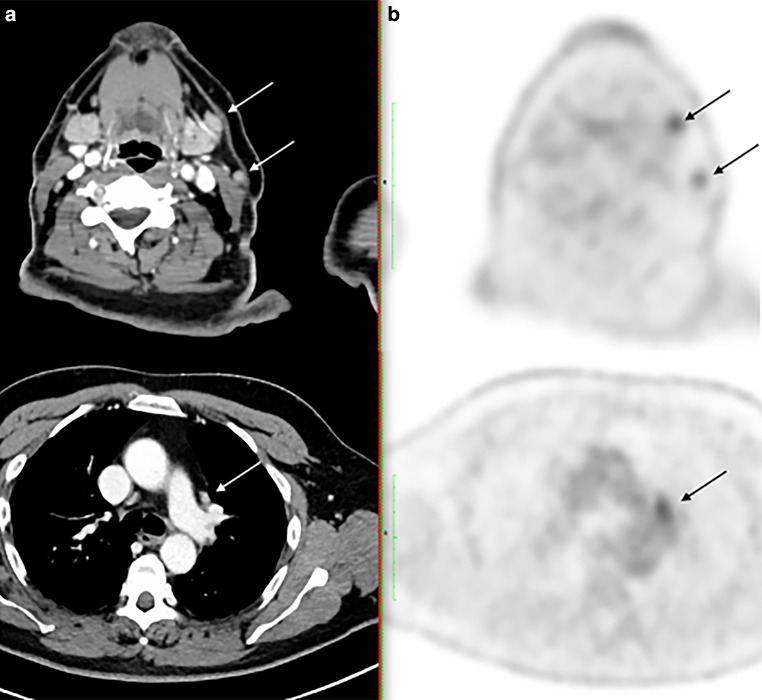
Assessment of CT morphology (a) and FDG uptake in PET (b) of lymph nodes in a patient with stage II follicular lymphoma. This particular patient had a lymph node resected to establish the diagnosis in the right cervical region. FDG-PET/CT led to extension of the radiation fields to include the left cervical (top; SUVmax: 7.0 and 4.4) and mediastinal region (bottom; SUVmax: 6.1). The arrows mark PET-positive lymph nodes. *CT* computed tomography; *PET* positron emission tomography; *FDG* ^18^F-Flourdesoxyglucose; *SUVmax* maximal standard uptake value
